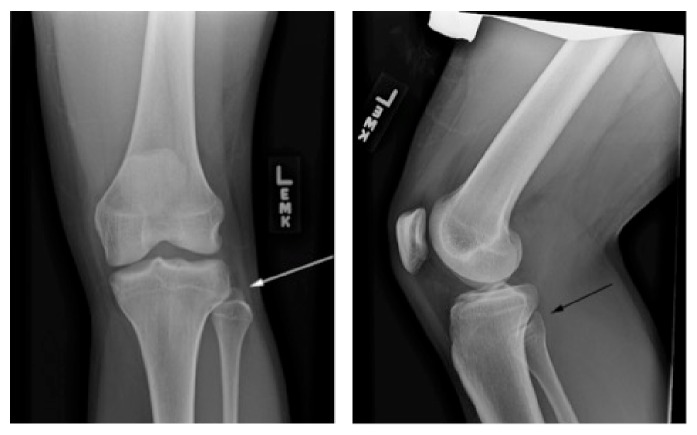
AP and lateral X-ray views of the left knee after reduction of the tibiofibular dislocation.
